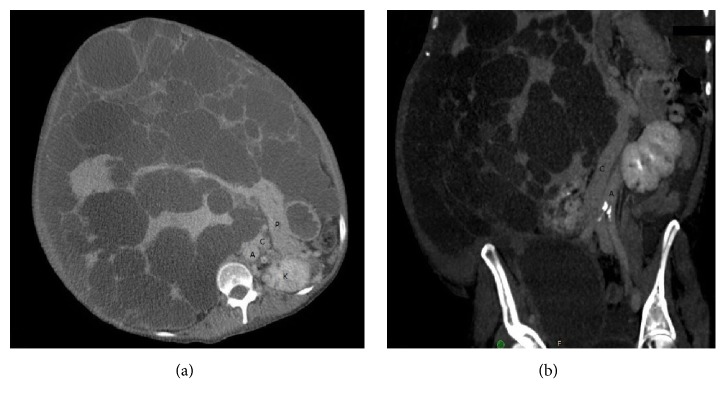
(a) Axial section CT showing abdominal anatomy with kidney (K) and pancreas (P) in relation to liver. (b) Coronal plane CT venogram showing vena cava (C) pushed on the left side of the aorta (A).
